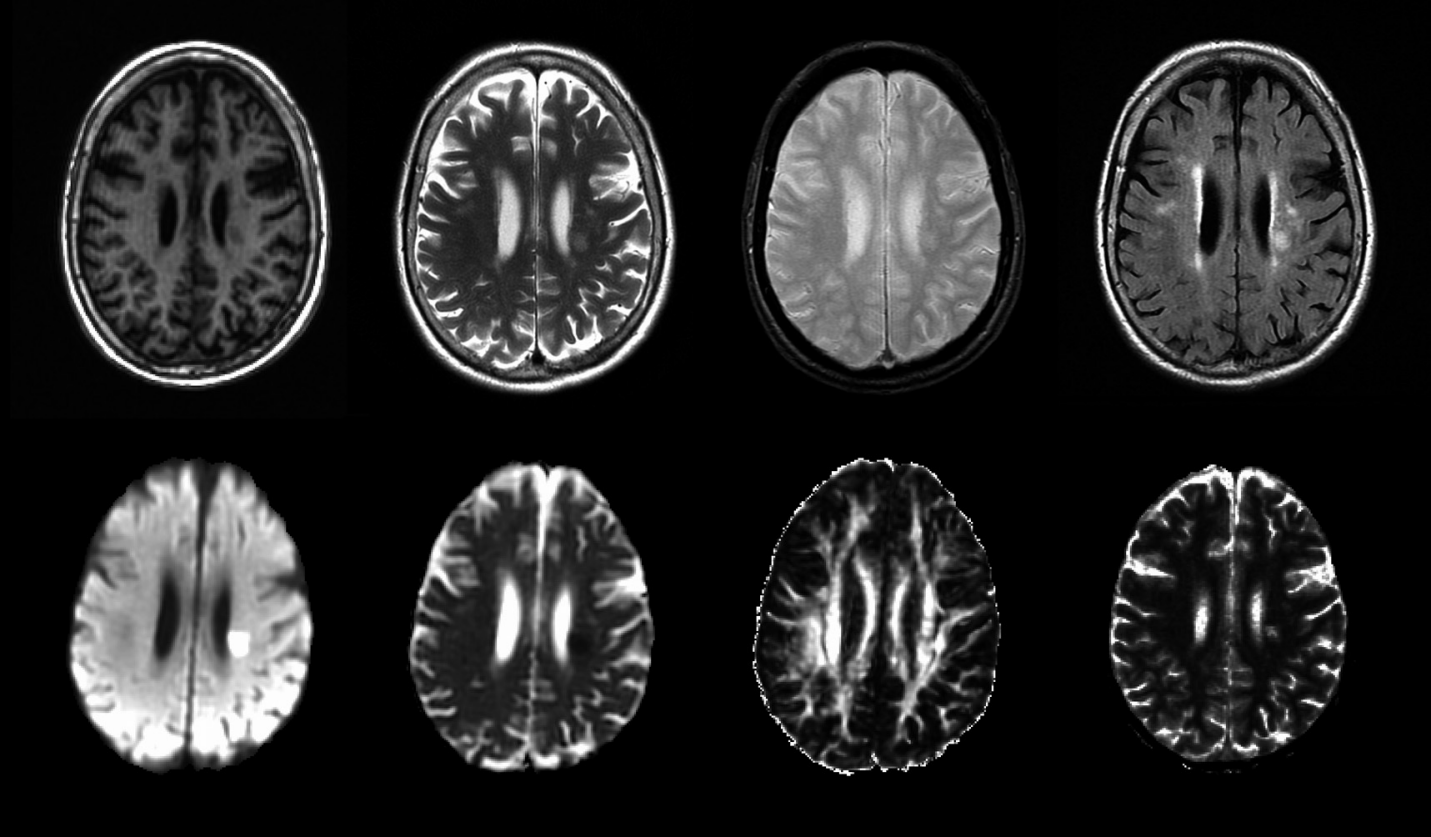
Axial slice from a patient showing the imaging modalities used in the multiparametric approach that this protocol describes. In the upper row, from left to right: structural T1‐weighted (for analyses of volumes and shapes), T2‐weighted (brain tissues, lesions, and perivascular spaces), gradient echo (ICV, brain tissues, lesions, mineral deposition, and microbleeds/hemorrhages) and FLAIR (brain tissues and lesions, especially WMH). In the bottom row, from left to right: diffusion‐weighted image (for identification of stroke lesions) and parametric maps of mean diffusivity, fractional anisotropy and longitudinal relaxation time (T1) (for quantitative tissue integrity analyses).
